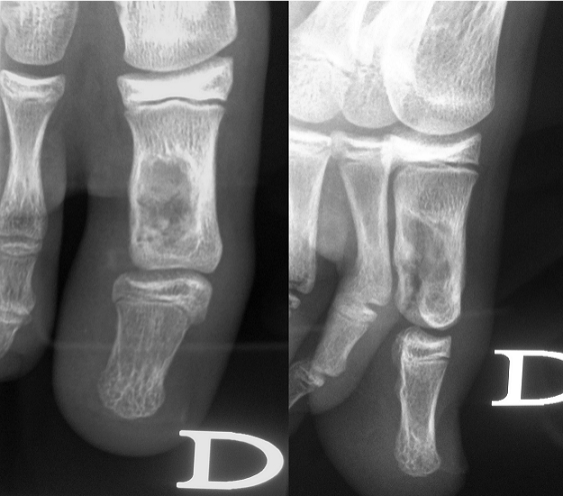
Anteroposterior and sagittal radiographs: well defined osteolytic lesion of the hallux proximal phalanx
